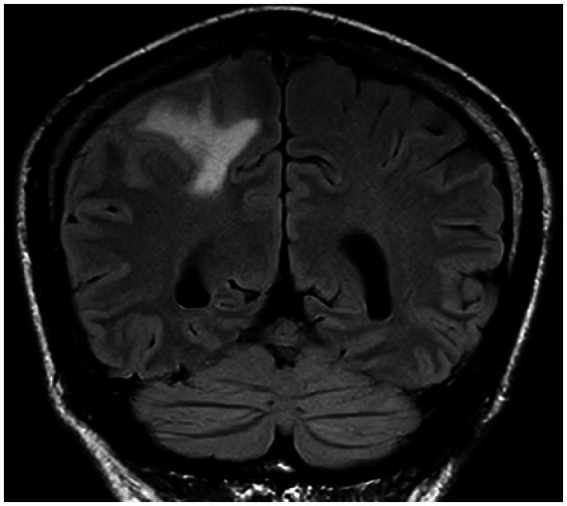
Signal alterations in the right parietal lobe (T2/FLAIR-hyperintense) suggestive of an infectious process.
